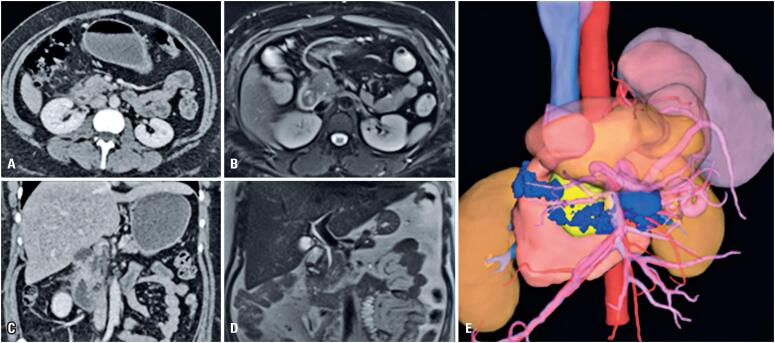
Portal phase of contrast enhanced abdominal CT, depicted in both axial (A) and coronal (C) views using the abdominal window. The images show irregular parietal thickening of the first and second portions of the duodenum, along with increased density of the surrounding fatty tissue. These findings are also evident in the corresponding abdominal MRI: the axial view with fat saturation using the T2 Blade sequence (B) and coronal view using the T2 Haste sequence (D). The 3D reconstruction of the CT (E), visualized in blue, reveals inflammatory tissue affecting the duodenum, leading to stenosis in the second portion and extending into the mesentery, which also causes stomach dilation. Pancreas is displayed in yellow
